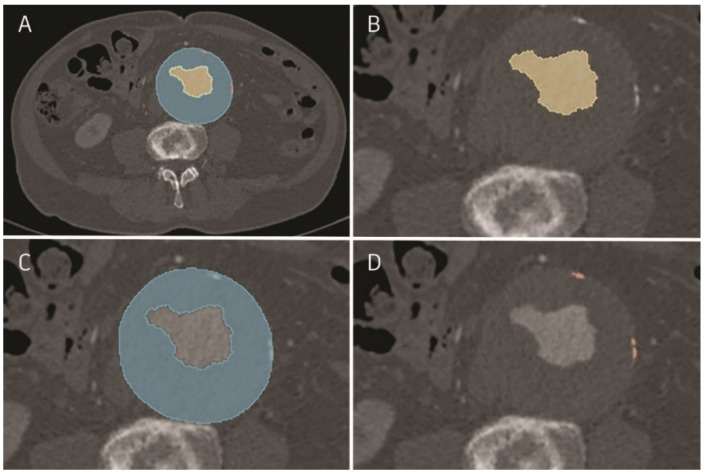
Semi-automatically obtained segmentation made in *3dSlicer* program: (A) computed tomography angiogram with segmented AFL, ILT, and calcifications; (B) aneurysm flow lumen (AFL) in AAA; (C) intraluminal thrombus (ILT) in AAA. Obtained region of ILT could contain calcifications; (D) calcifications in AAA.
